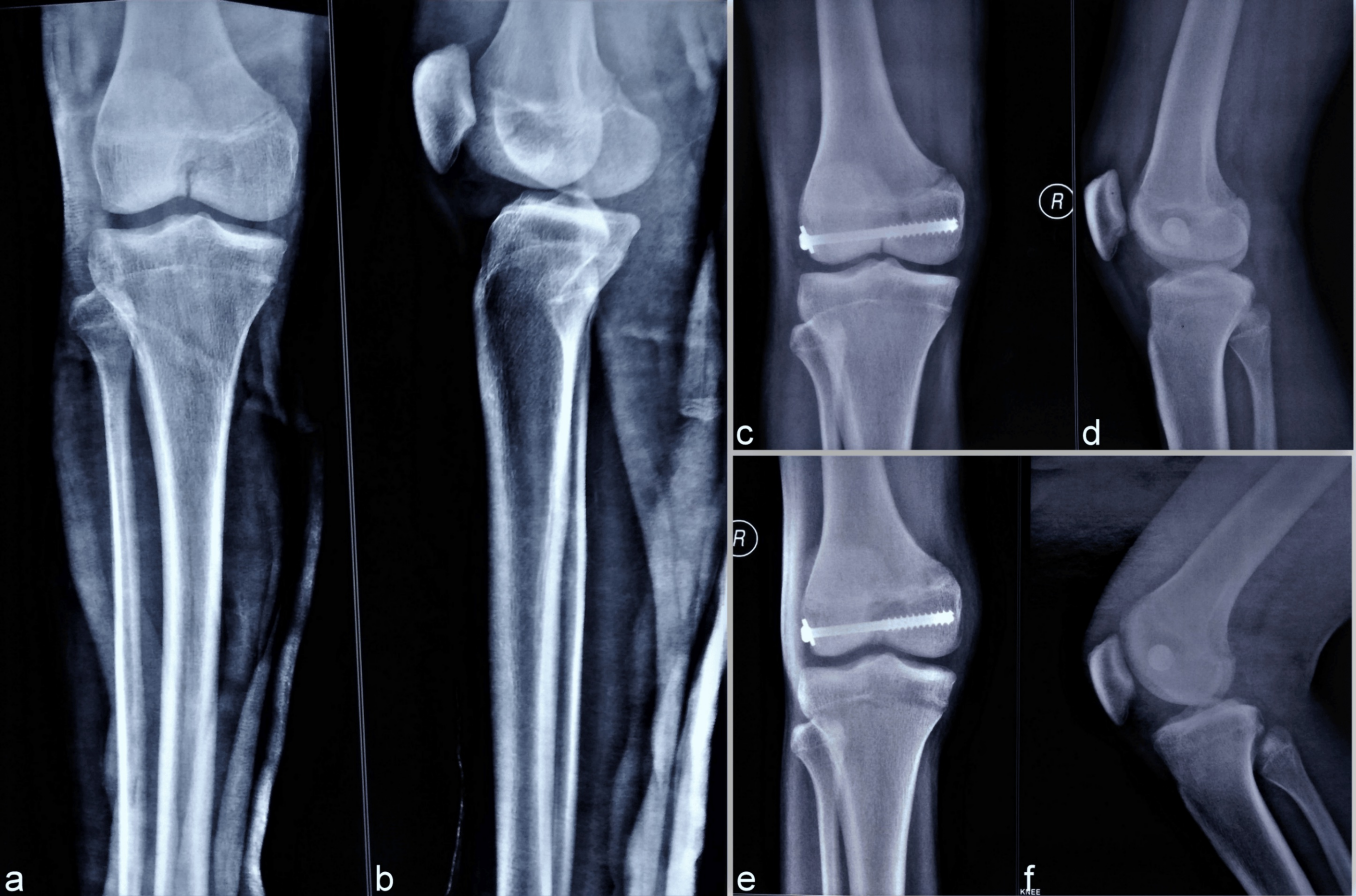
Radiographs show the presence of an undisplaced, incomplete fracture noted in the intercondylar distal femur area, both in the anteroposterior (a) and lateral (b) views. The fracture is not extending into either condyle. The fracture was fixed with a percutaneous screw fixation and showed good placement in anterioposterior (c) and lateral (d) views. Good union was achieved in the follow-up at 11 months in orthogonal (e, f) views with stable knee and full range of motion.
